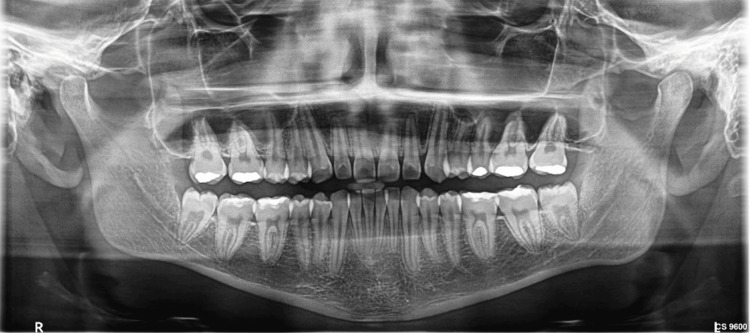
An orthopantomogram showing nerve involvement
